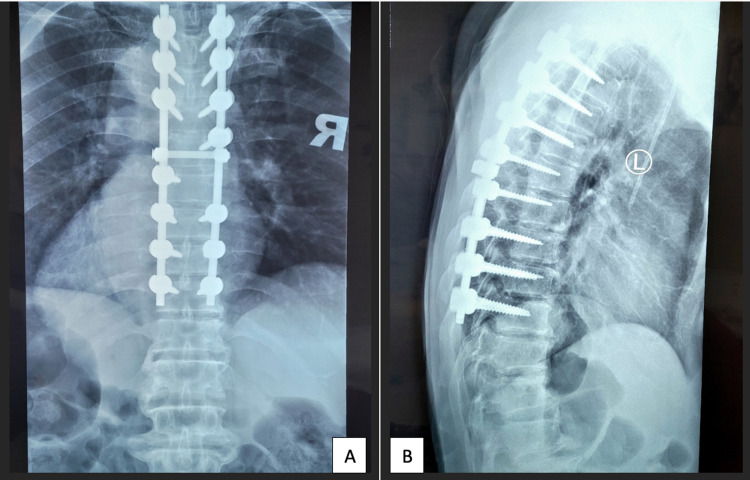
Postoperative 1-month check radiographs: (A) Anteroposterior view. (B) Lateral view.
